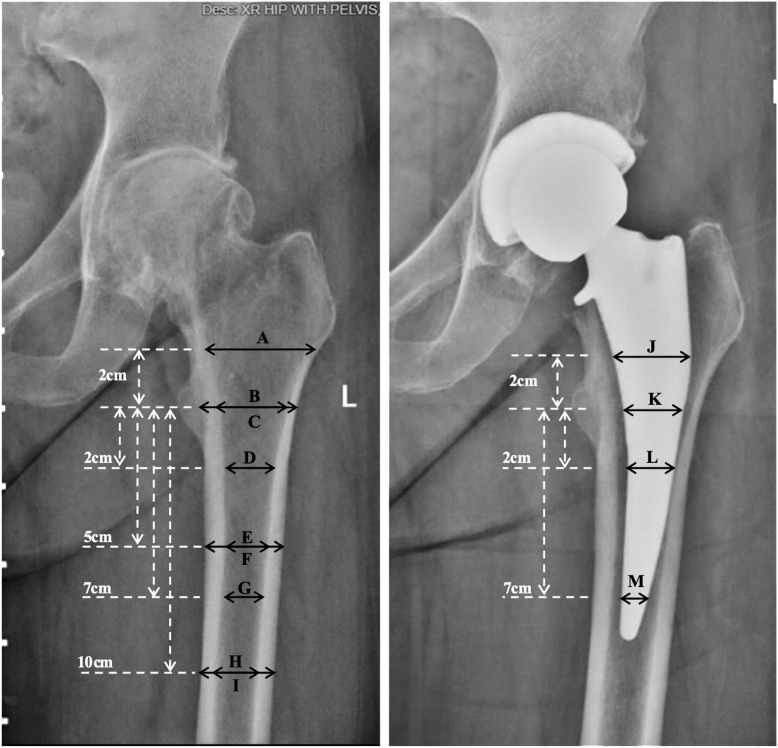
Radiographic measurements of proximal femur morphology preoperatively and stem canal fill ratio postoperatively.
